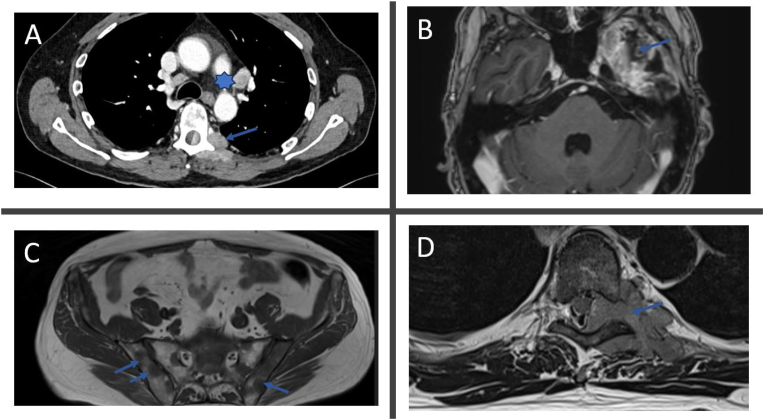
Recurrence. MRI and CT from intra and extracranial progression (A) Transversal CT with contrast shows a large contrast-enhancing paravertebral soft tissue lesion at the left side in the level with Th6. The tumor is growing through the neuroforamina, measuring 5 x 3 cm. Star shows pathological lymph nodes. Arrow shows paravertebral metastasis. (B) Transversal T1 with contrast shows progression of residual tumor tissue basomedial in the temporal fossa. (C) Transversal T1 weighted MR shows multiple hypointense metastatic lesions in the skeleton (sacrum and ilium). Arrows show metastasis. (D) Transversal T2 weighted MRI of the same soft tissue lesion as A. Arrow shows paravertebral metastasis.
